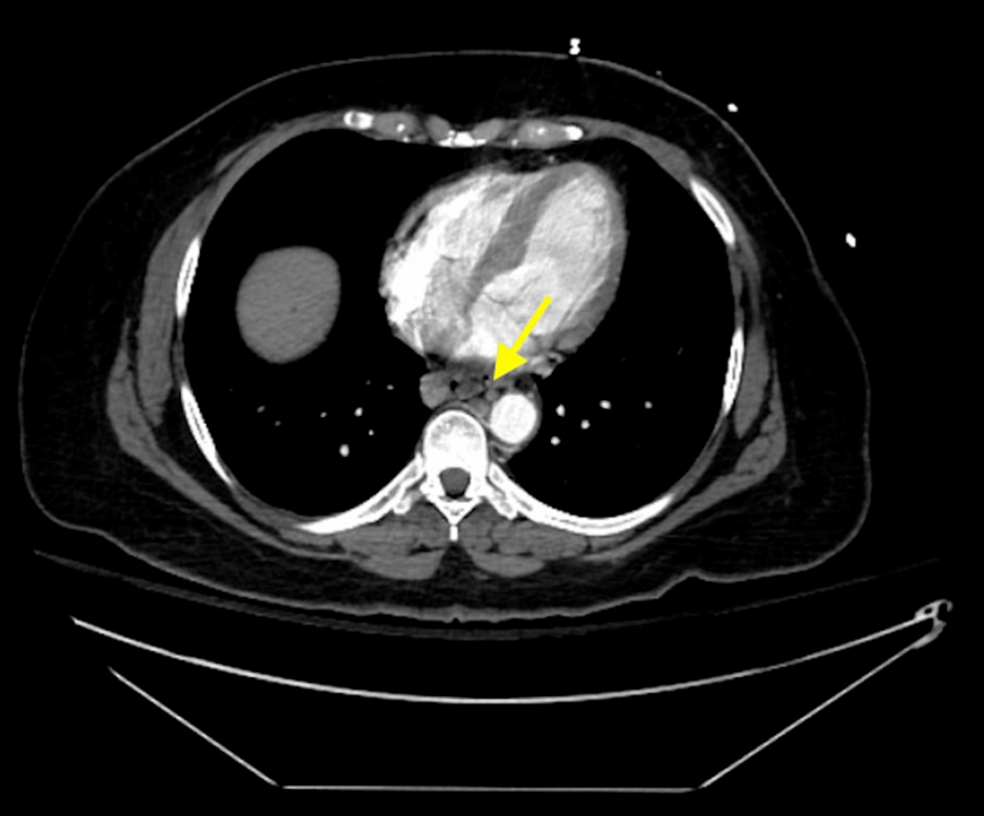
CT angiography of the abdomen and pelvis with and without contrast demonstrating lower esophageal varices.
